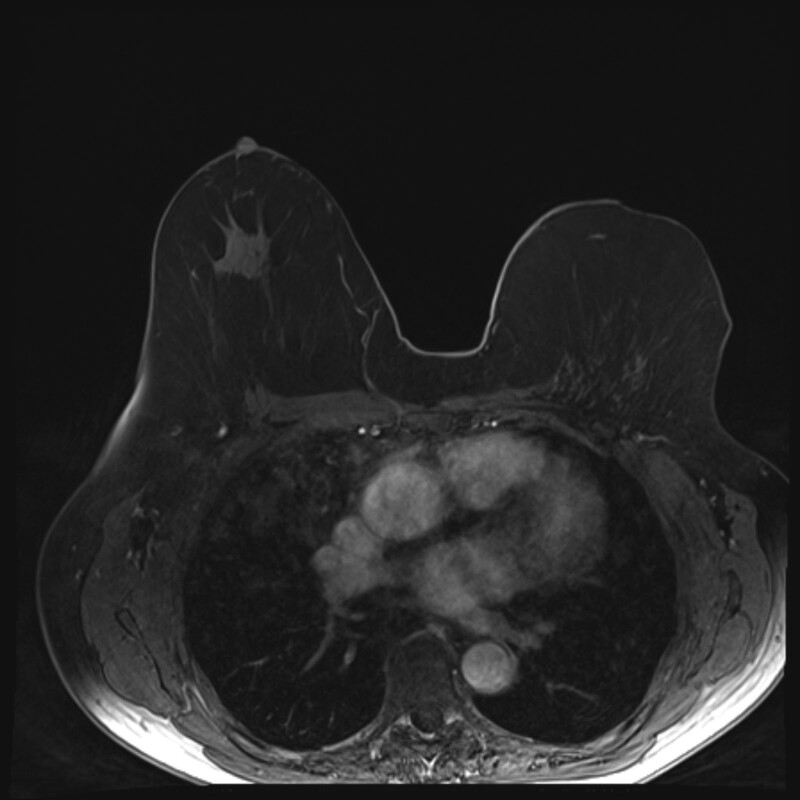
A T1-weighted MRI scan of the breasts of patient 2 from Figure 2, 8 years after the reconstruction of the left breast with four lipofilling operations.
